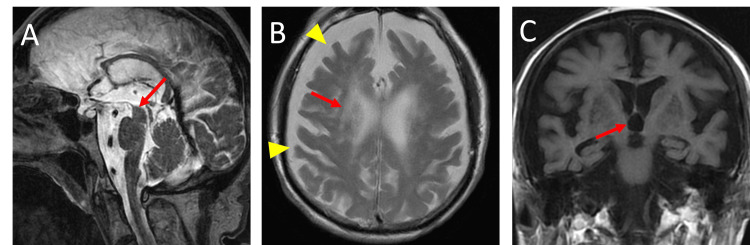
Brain magnetic resonance imaging of patient 1 (A) T2-weighted sagittal image shows midbrain atrophy (arrow). (B) T2-weighted horizontal image shows diffuse cortical atrophy (arrowheads) with an ischemic change in deep white matter (arrow). (C) T1-weighted coronal image shows the dilation of the third ventricle (arrow).
